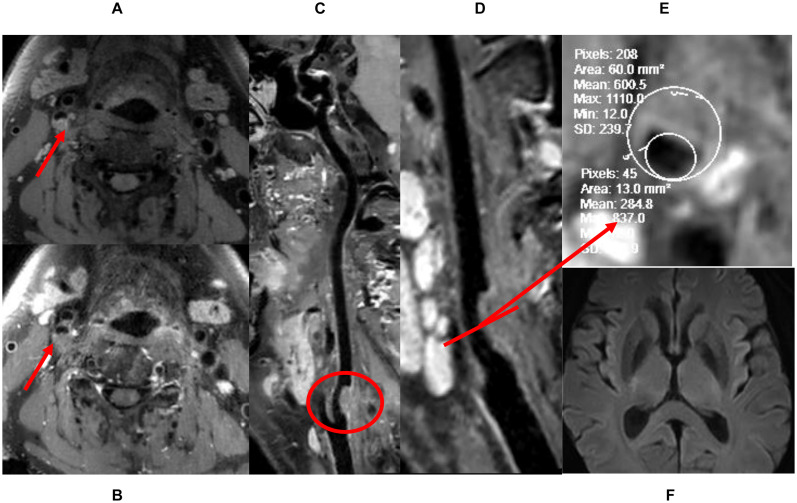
The definition of low-risk plaque. (A) Pre-enhanced HRVW 3D T1 image. Intensity is homogeneous was seen in the plaque. (B) Post-enhanced HRVW 3D T1 image. No abnormal intensity was seen in the plaque. (C,D) Reconstruction map of post-enhanced HRVW 3D T1 image. Better display of the whole vessel and the plaque shown in red circle. (E) Section image, perpendicular to the lumen located in the plaque lesion. The stenosis rate can be determined in this section. (F) DWI. No abnormal hyperintensity was found in the whole brain, indicating no cerebrovascular events occurred.
